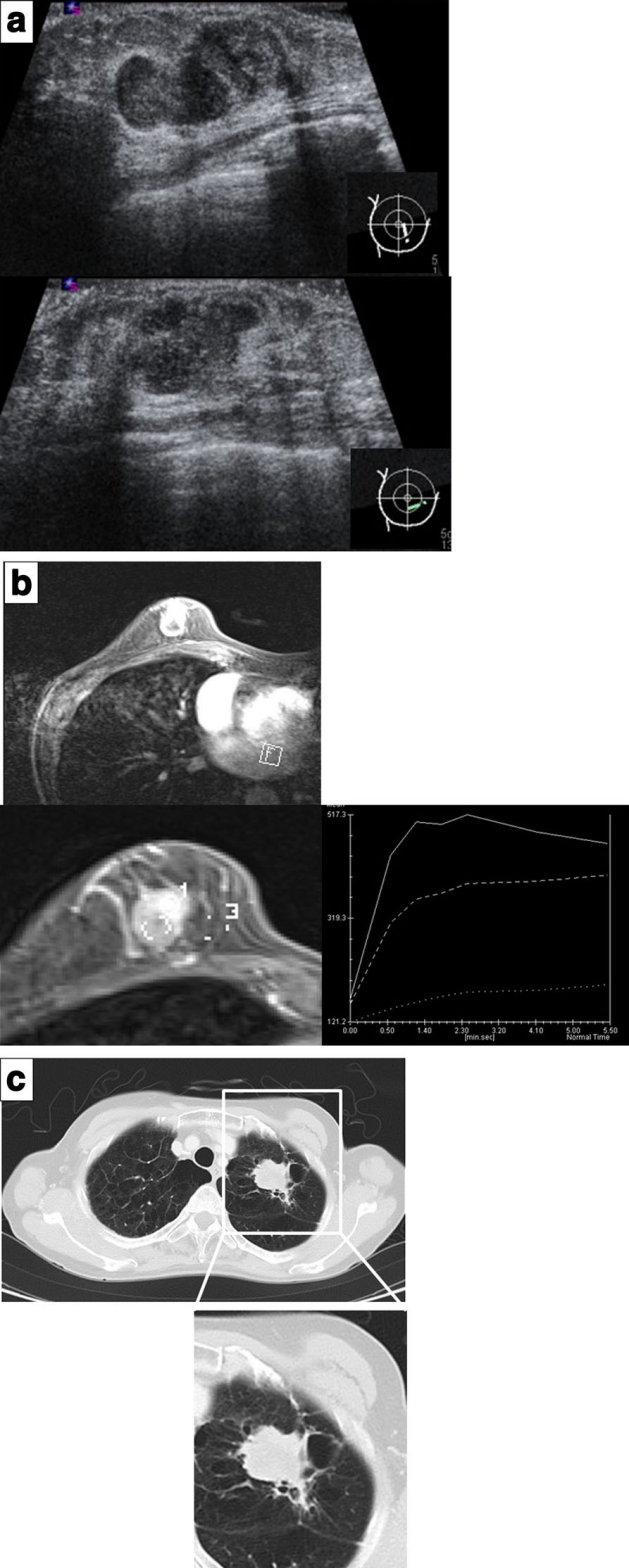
a On mammary ultrasonography, an extensive lobular lesion of 12 × 24 × 17 mm with a clear coarse boundary was observed in the right B/D region. b On mammary MRI, a contrast-enhanced mass was observed from the early phase. c On chest CT, an irregular tumor that was suspected to be lung cancer was present in the left upper lobe
